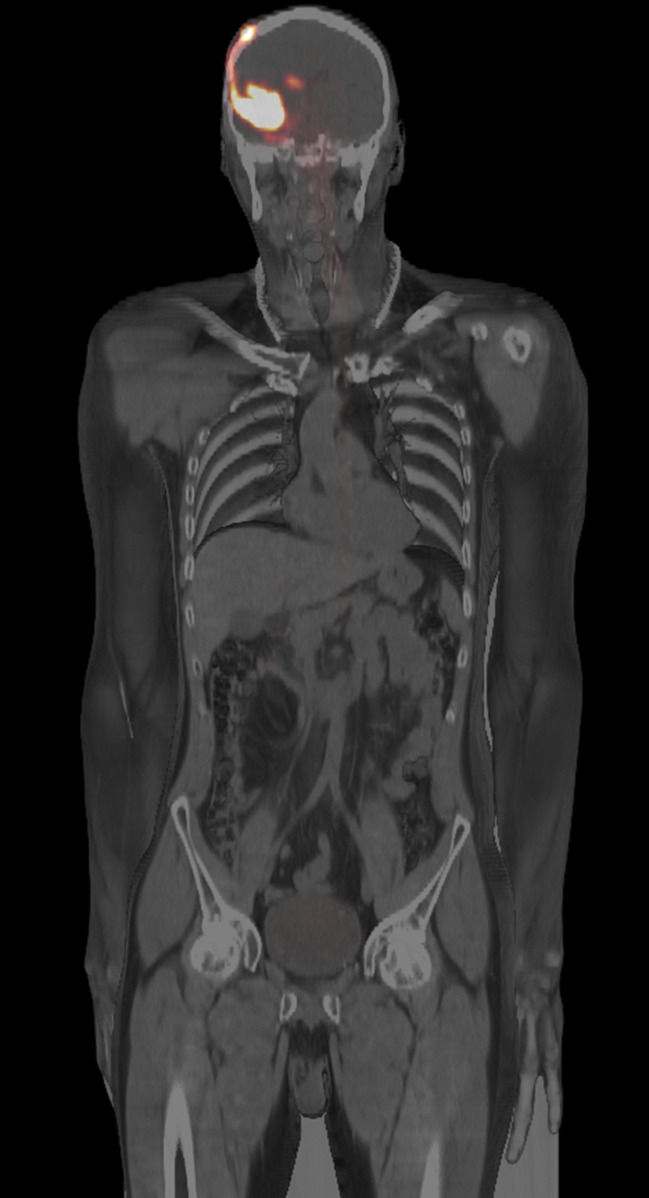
Whole body PET/CT scan shows biodistribution 30 min after intralesional injection of 10 MBq ^68^Ga-DOTA-SP analogue: the signal detected in the body outside the brain is very faint or negligible in liver, kidney, spleen and bone marrow. The cleaved linear peptidic vector is excreted into the bladder and can show a weak signal corresponding to <5% of injected activity
